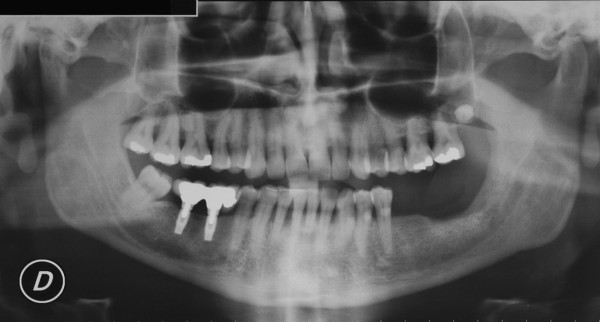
panoramic radiograph of patient n°2. Note the erosion of the bone on the left side of the mandible.
